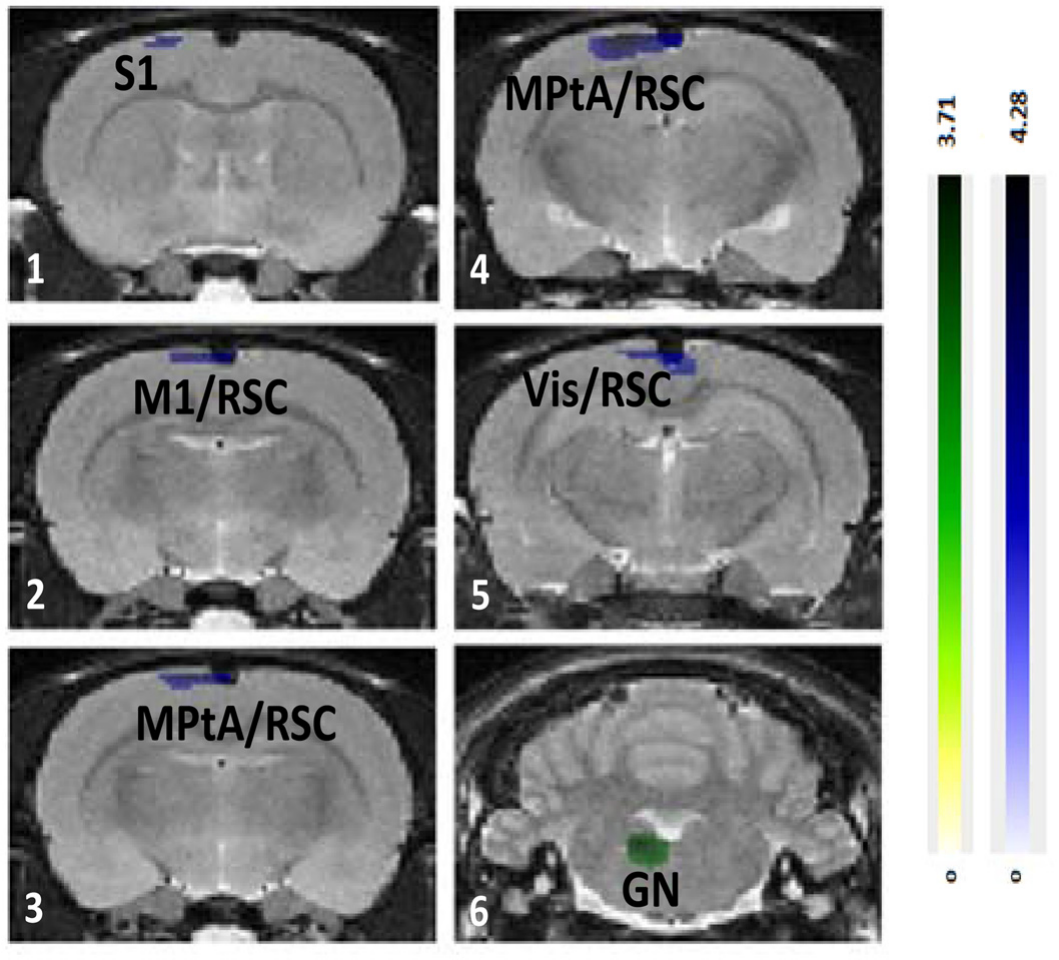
Differential effects of 4 and 8 mg/kg MA treatments on regional brain glucose metabolism. Regional brain glucose metabolism differences between 4 and 8 mg/kg MA treatments, superimposed on the Schweinhardt MRI template, in Paxinos space. Green: brain regions with increased glucose metabolism after 4mg/kg MA treatment compared with 8 mg/kg MA treatment. Blue: brain regions with decreased glucose metabolism after 4mg/kg MA treatment compared with 8 mg/kg MA treatment. S1: primary somatosensory cortex; M1: primary motor cortex; RSC: retrosplenial cortex; PtA: parietal association area; Vis: visual cortex; RSC: retrosplenial cortex; GN: gigantocellular nucleus.
